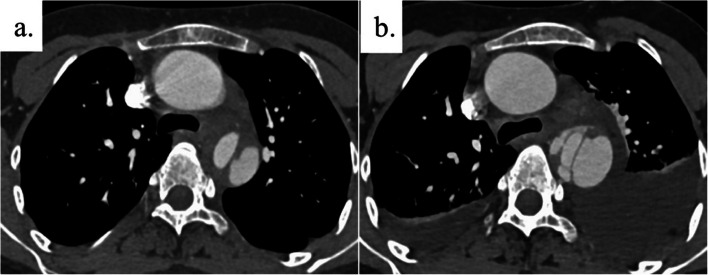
a The initial CT scan upon admission showed Stanford type B acute aortic dissection. The diameter of the descending aorta was 36 × 34 mm. b Follow-up CT scan on day 5 after the onset revealed a three-channel aortic dissection in the descending aorta, an increased pleural effusion, and a dilated descending aorta (39 × 36 mm)
